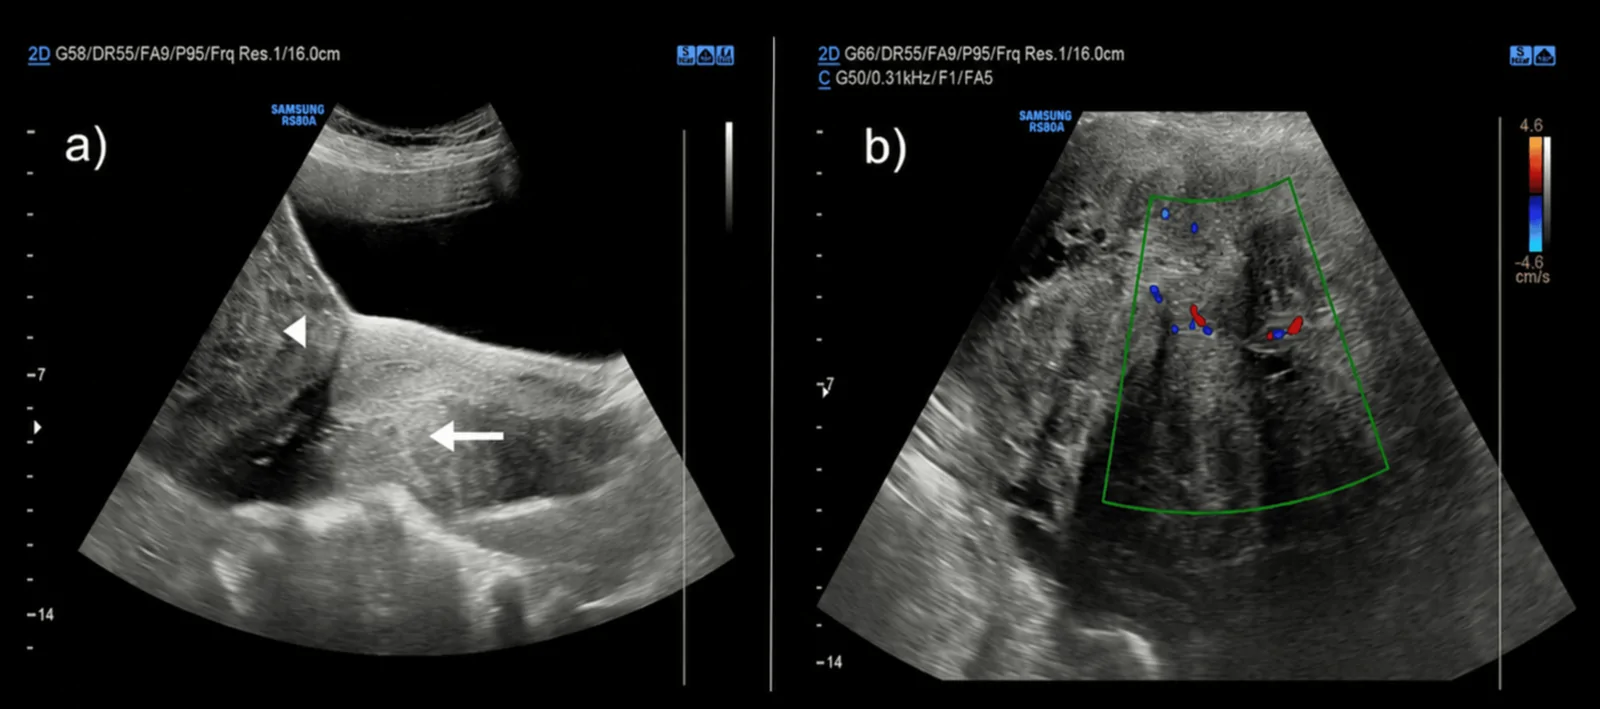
Ultrasonography findings (a) Gray-scale ultrasound image showing a large, heterogeneous, predominantly solid abdominopelvic mass with internal cystic areas adjacent to the uterus (arrowhead), with the uterus seen separately from this lesion, indicated by the arrow. (b) Color Doppler image demonstrating mild internal vascularity within the predominantly solid mass.
